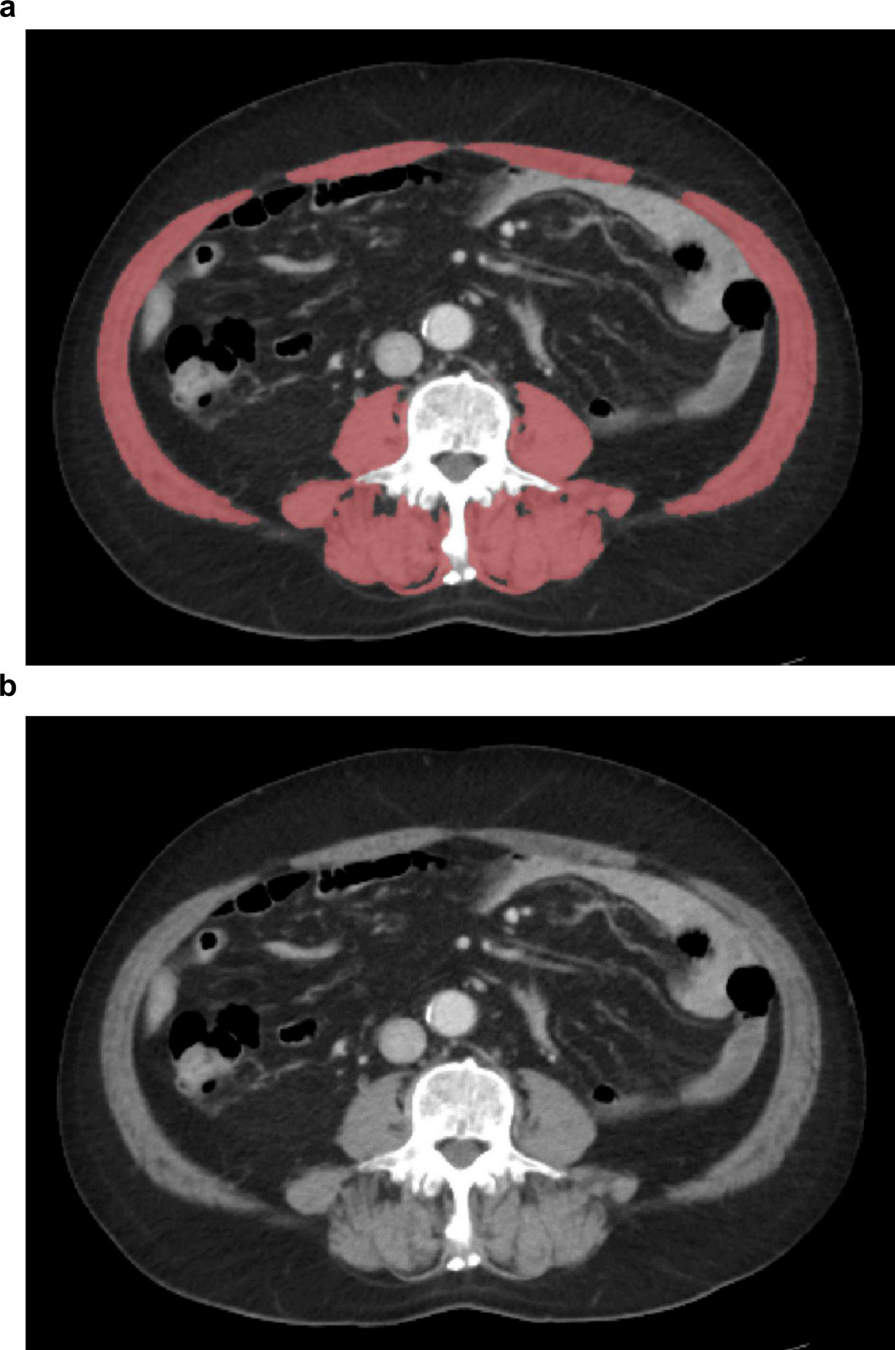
Automated segmentation of skeletal muscles. (a) Axial postcontrast computed tomography (CT) image at the third lumbar vertebra level in a 70‐year‐old male with a BMI of 28.9 kg/m^2^. (b) Visualization of segmented skeletal muscles highlighted in red overlays. SMI = 49.35 cm^2^/m^2^ and SMD = 24.21 HU, indicating myosteatosis in the patient. BMI, body mass index; HU, Hounsfield unit; SMI, skeletal muscle index; SMD, skeletal muscle density.
